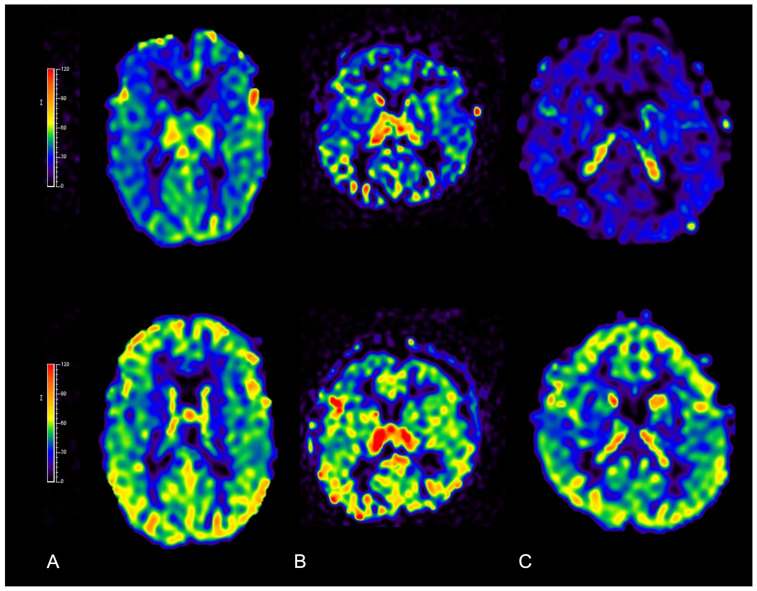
Qualitative increase in cerebral blood flow demonstrated with ASL perfusion MRI before (upper row) and 6 months after surgery (bottom row) in three patients diagnosed with nonsyndromic isolated CRS: (A) scaphocephaly; (B) anterior plagiocephaly; and (C) trigonocephaly. Threshold color scale bars are shown on the left.
